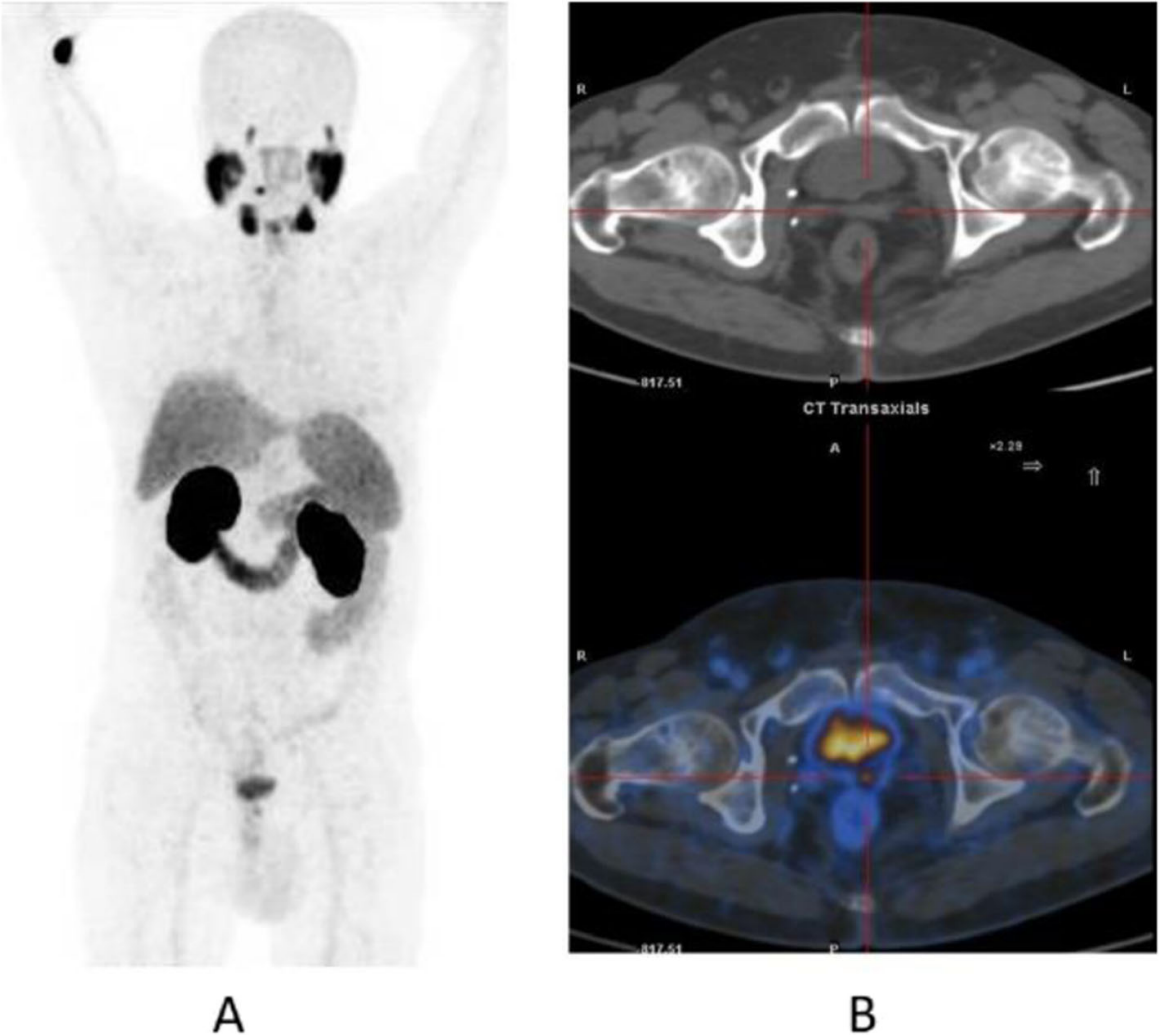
^68^Ga-PSMA, prostate cancer, biochemical recurrence (BCR). *Clinical history*: 62 y.o. man with prostate cancer, radical prostatectomy as primary treatment Gleason score 4 + 4; PSA = 0.7 ng/ml, PSAdt 5 months, TTR 24 months, candidate to salvage radiation therapy to the prostatic fossa. *PET/CT findings*: single focus of increased uptake seen in the prostatic fossa consistent with local relapse after biopsy. a MIP; b CT and fused images
